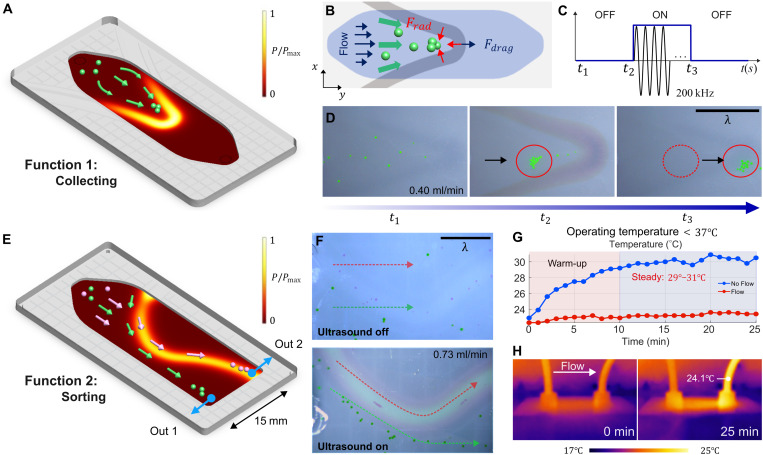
Advanced functionalities enabled by the acoustic virtual wall. (A) Collector design for PS particles. The acoustic virtual wall is configured to collect PS particles by balancing the radiation force against drag from the flow. (B) Collection mechanism. The radiation force traps incoming particles near the virtual wall, where they accumulate until release. (C) Programmable collection with automatic on-off control. Ultrasound activation at $t_{2}$ enables particle collection, and deactivation at $t_{3}$ releases the accumulated particles. (D) Experimental demonstration. PS particles converge to the channel center during ultrasound excitation at $t_{2}$, where they accumulate. Upon deactivation at $t_{3}$, the aggregated particles are released and advected downstream. (E) Sorter design for PS and PDMS particles. The acoustic virtual wall is engineered such that PS and PDMS particles experience different signs of acoustic radiation force, enabling separation at two distinct outlets. (F) Experimental results of sorter design. Under a flow rate of 0.73 ml/min, PS particles are expelled from the virtual wall and carried to outlet 1, while PDMS particles are guided along the virtual wall toward outlet 2. (G) Measured temperature of the platform during ultrasound excitation with and without the flow. The device temperature increases during ultrasound excitation and reaches steady state between 29° and 31°C without the flow and 24°C with the flow. (H) Surface temperature of the platform with the flow. The operating temperature remains below 37°C, within typical limits for biological applications.
